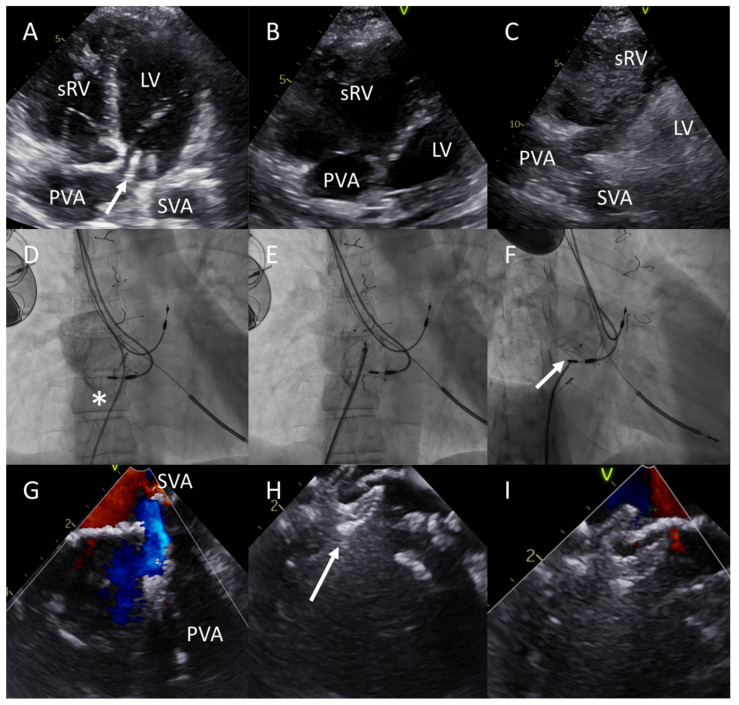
Baffle leak visualisation and closure in patient A. (A,B) Apical four chamber and subcostal views, respectively show the atrial baffle (arrow) that directs blood from the systemic venous atrium (SVA) to the subpulmonary left ventricle (LV) and from the pulmonary venous atrium (PVA) to the dilated systemic right ventricle (sRV). (C) Agitated saline contrast echocardiography confirms significant interatrial shunting from the SVA to the PVA during Valsalva, with bubbles filling the sRV cavity within 3 heart beats. (D–F) Anterior-posterior (D,E) and right inferior oblique (F) fluoroscopy projections during intracardiac echocardiography (ICE, probe indicated with an asterisk (*) in (D)) guided closure of the baffle leak with a 10 mm Amplatzer Septal Occluder (arrow in F). Note the two atrial pacing wires and an implantable cardioverter-defibrillator (ICD) lead in the LV. (G–I) ICE imaging during closure of the baffle leak. Note the shunting from the SVA to the PVA during Valsalva through the baffle defect (G), the discs of the Amplatzer Septal Occluder in situ (H, arrow) and the alleviation of the interatrial shunt (I).
